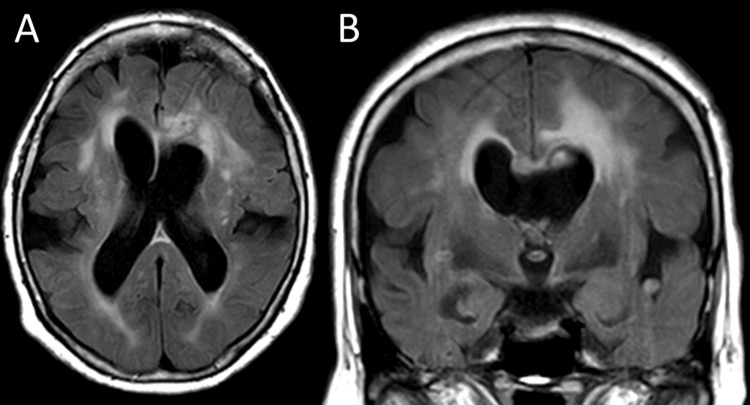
Postoperative MRI. The tumor at the septum pellucidum was removed (A, B).
